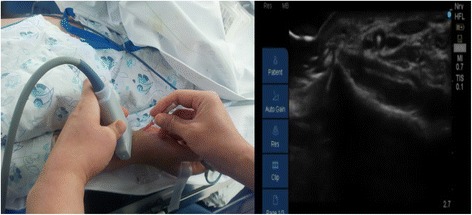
The puncture needle is inserted perpendicular to the transducer (left image) and appears on theultrasound screen (right image) as a dot within the lumen of the radial artery
